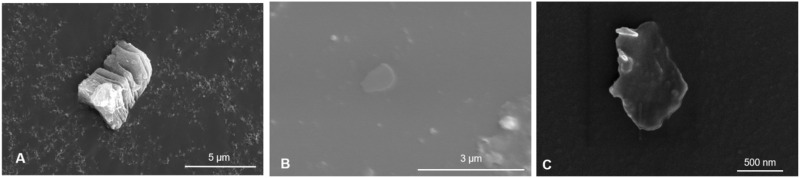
Multi-layer clay Ti_3_C_2_Tx MXene SEM image (A). Ultrasonic dispersed few/single layer Ti_3_C_2_Tx MXene SEM image (B, C).
